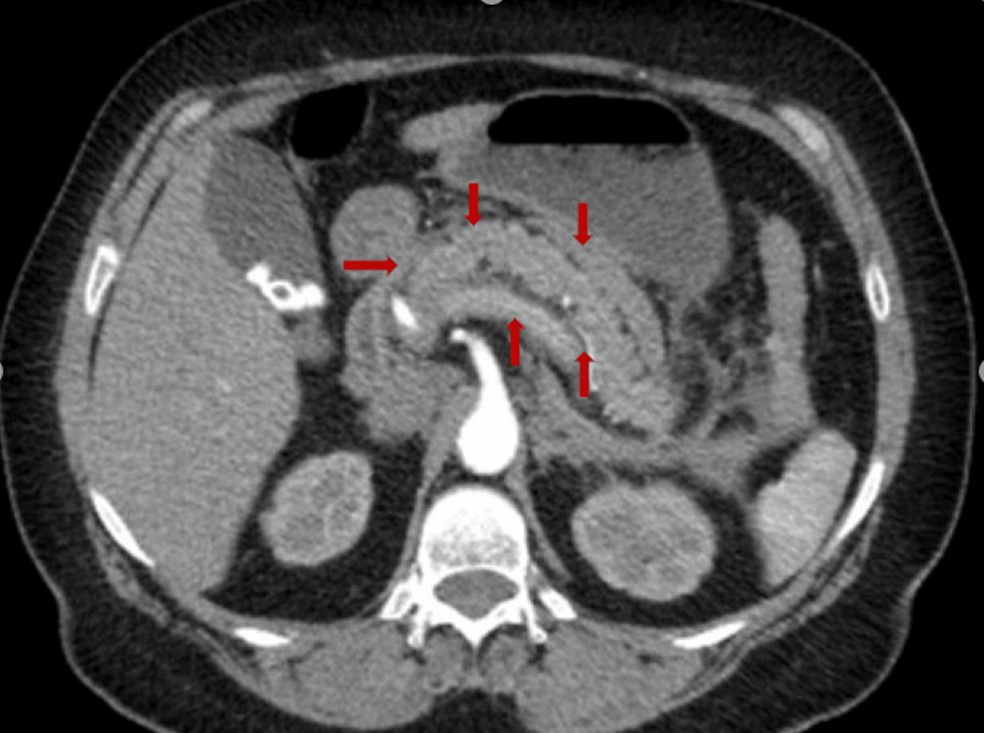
Computed tomography angiography (CTA) of the abdomen showing diffuse pancreatic enlargement with edema (red arrows).
